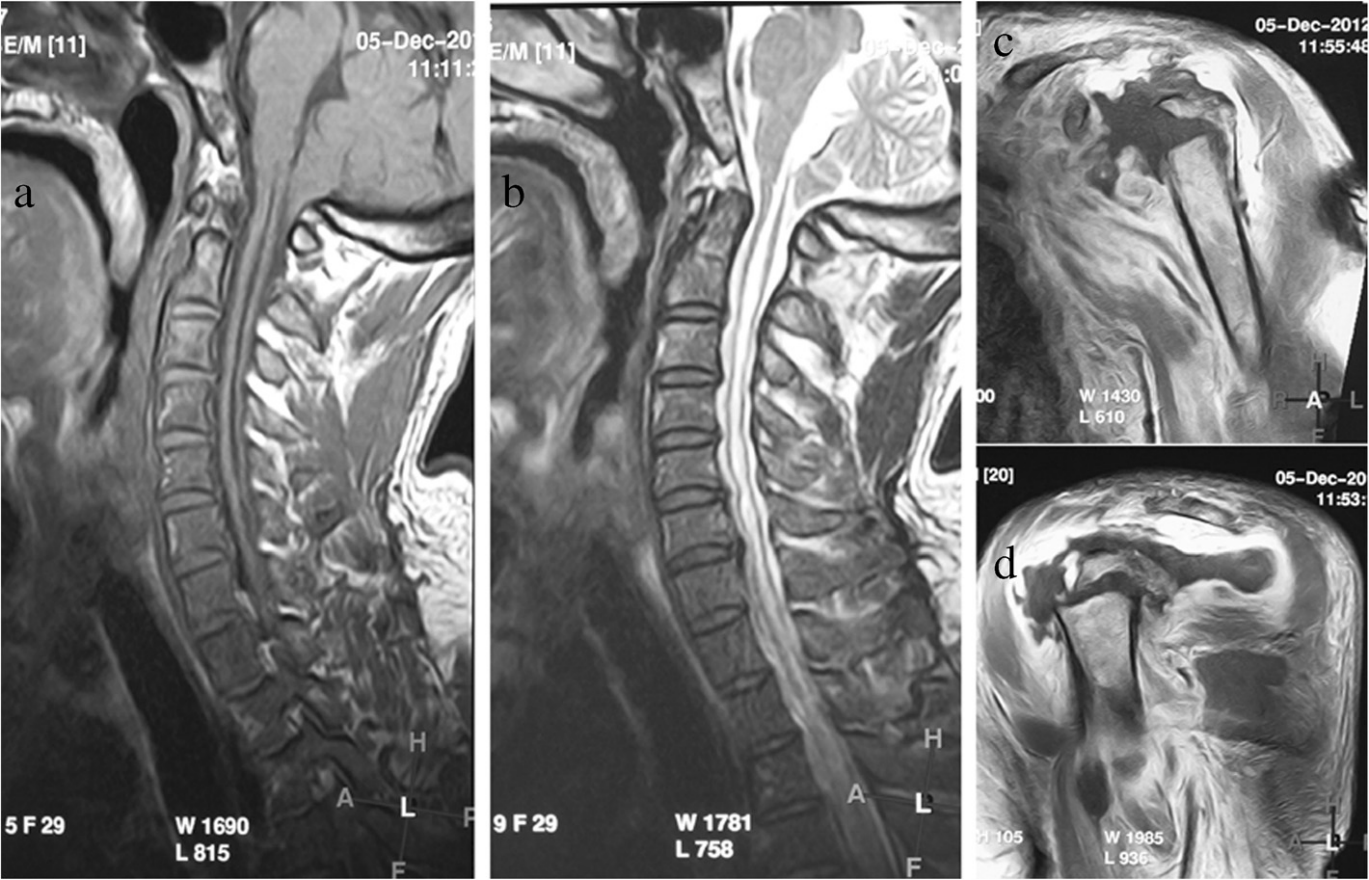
MRI of this case. A significant cerebellar tonsillar hernia (a), Syringomyelia from C1 to T4 (b), disappearance of humeral head and swollen soft tissue of the surrounding of the joint (c and d).
